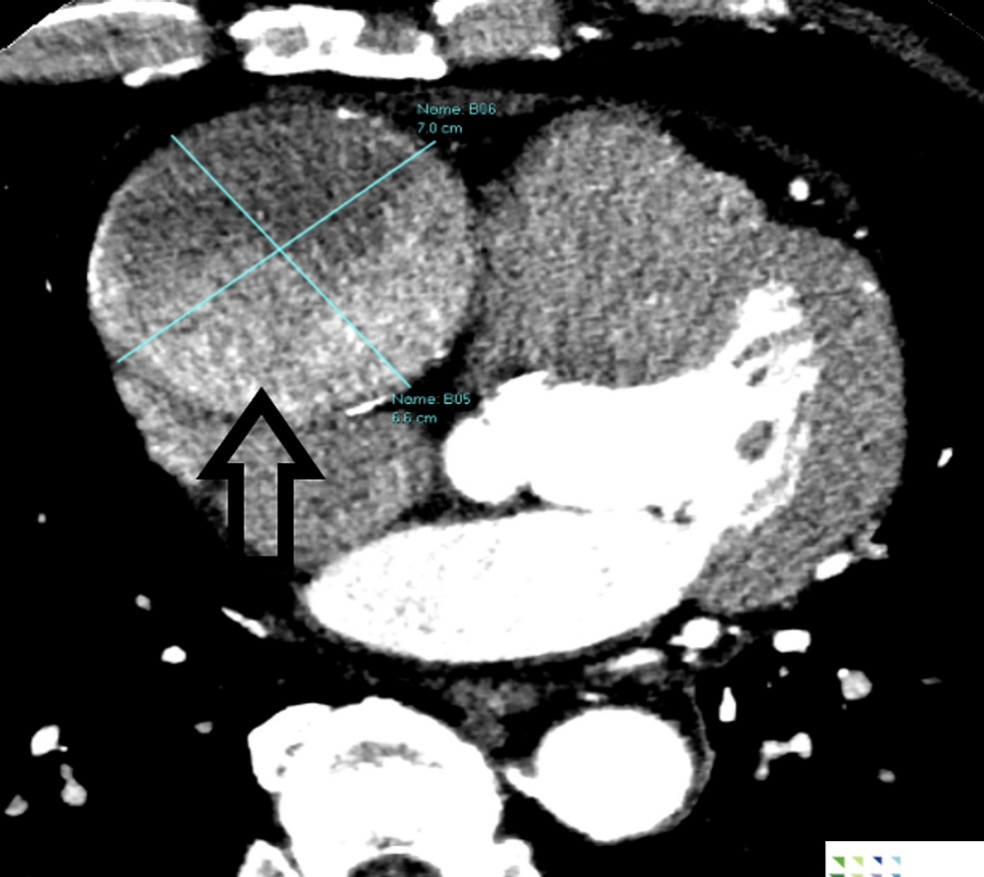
Dual-source CT showing the giant aneurysm (9.0 x 7.5 cm) and the affected vessel.
